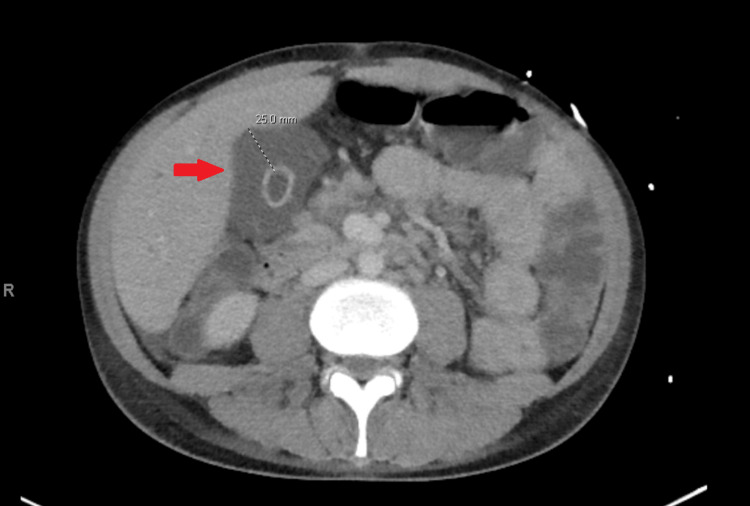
CT abdomen with contrast shows significant gallbladder mucosal thickening and edema. Gallbladder wall measures 25 mm in thickness (red arrow), without evidence of cholelithiasis.
